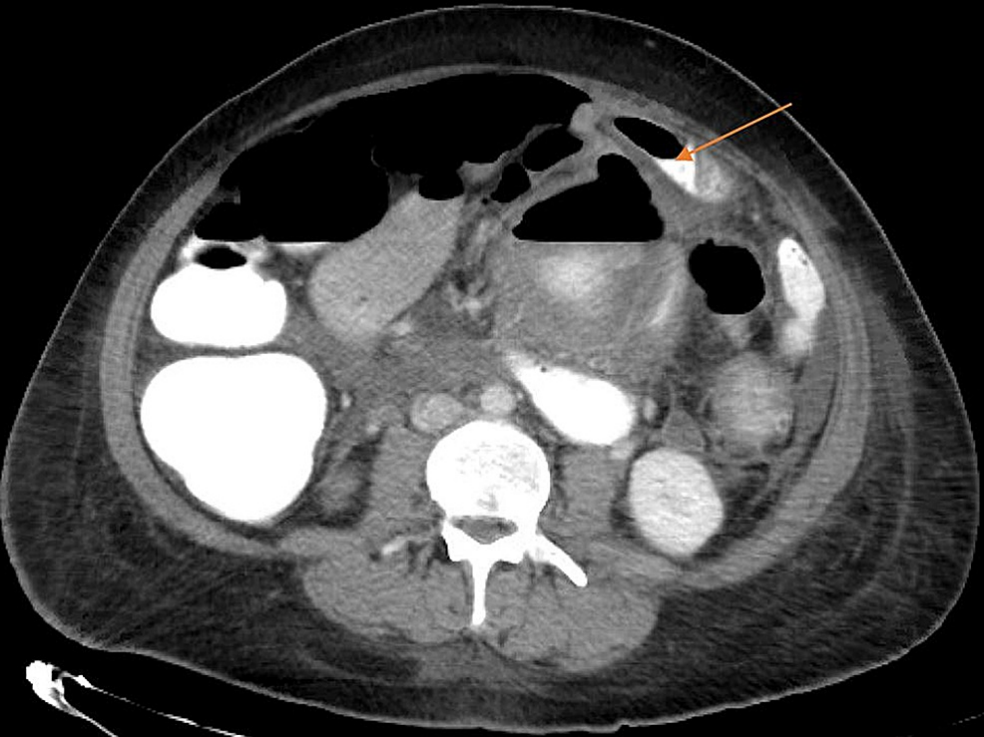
CT abdomen/pelvis with oral contrast (axial cut) showing a large amount pneunoperitoneum and extravasation of contrast in the mid-descending colon (orange arrow)
